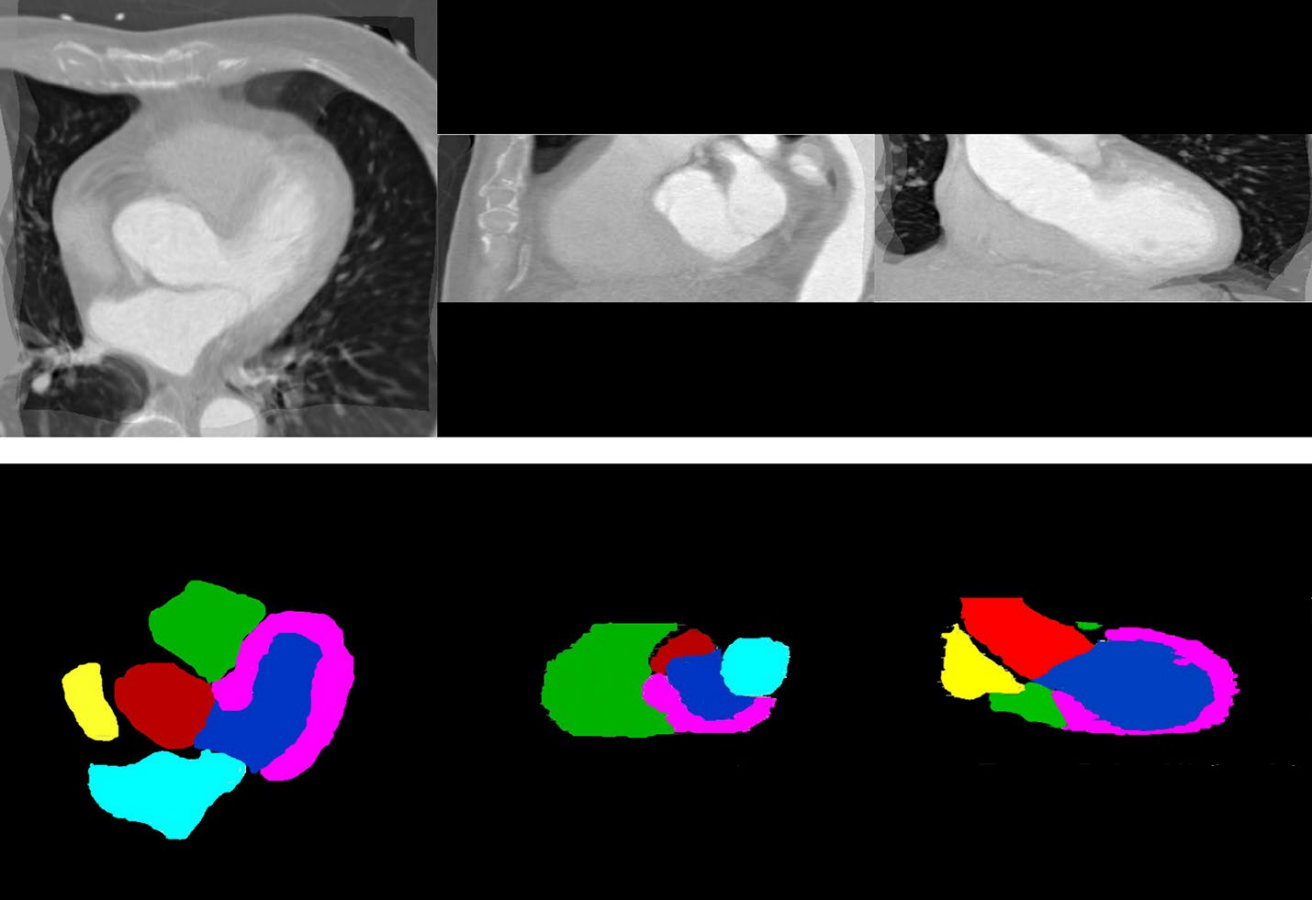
An atlas example that is shown in axial, sagittal, and coronal planes. *Upper* an atlas intensity (CT) image using the mean shape of ten patient data. *Lower* atlas label image including aorta (*crimson*), left atrium (*cyan*), left ventricle (*blue*), left ventricle myocardium (*magenta*), right atrium (*yellow*), and right ventricle (*green*)
